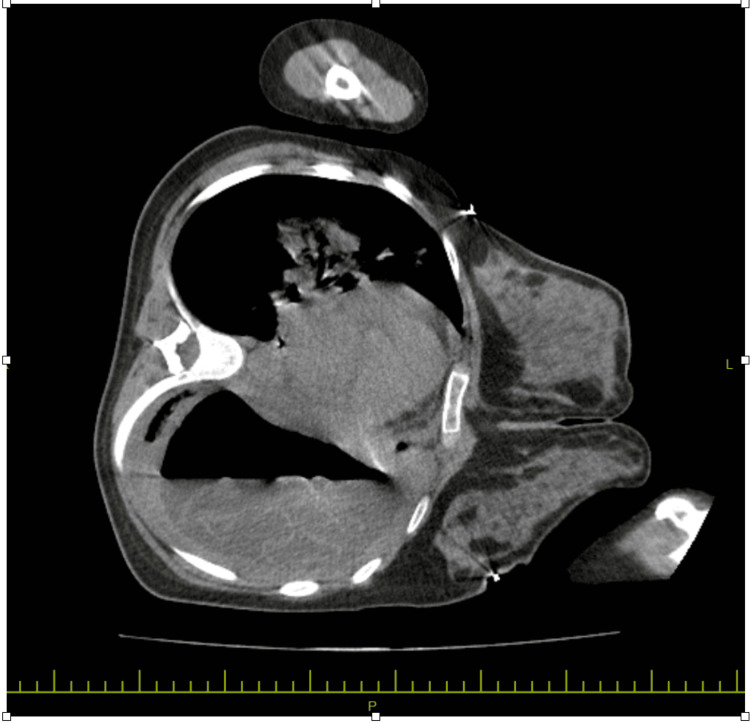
CT scan of the chest (mediastinal window) A giant cyst measuring 11 × 16 cm with a thick wall and floating hydatid membranes in the pleural fluid (serpent sign).
